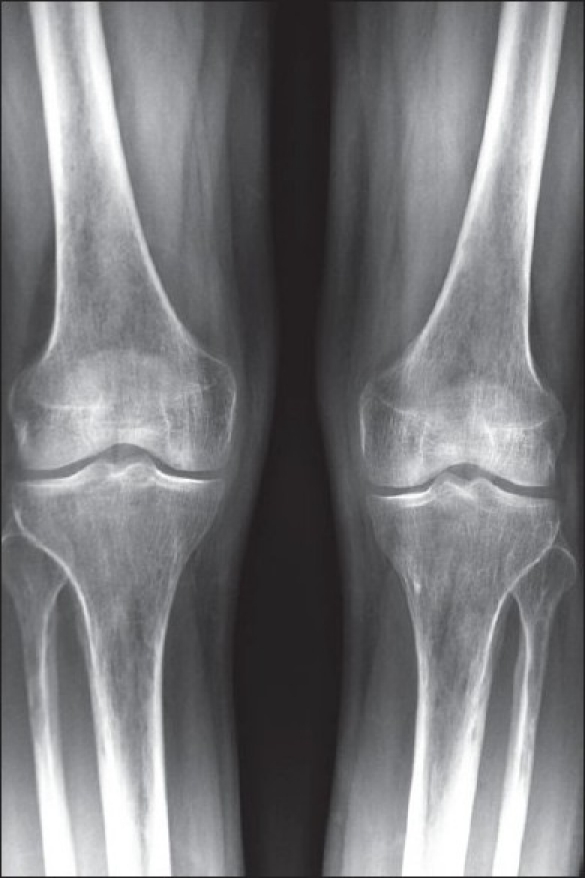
X-ray of both knees showing osteoporosis
